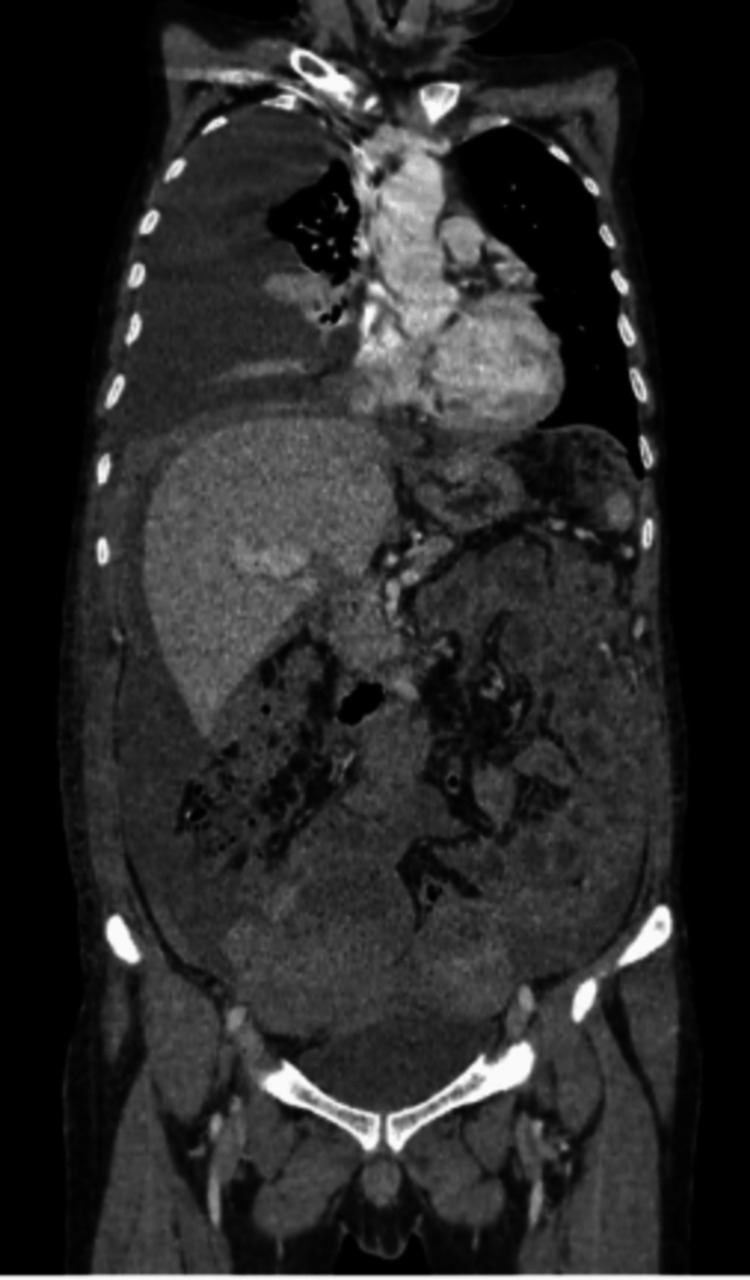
Coronal image of a CT scan showing around 15×15 cm mass in the pelvis CT: computed tomography
